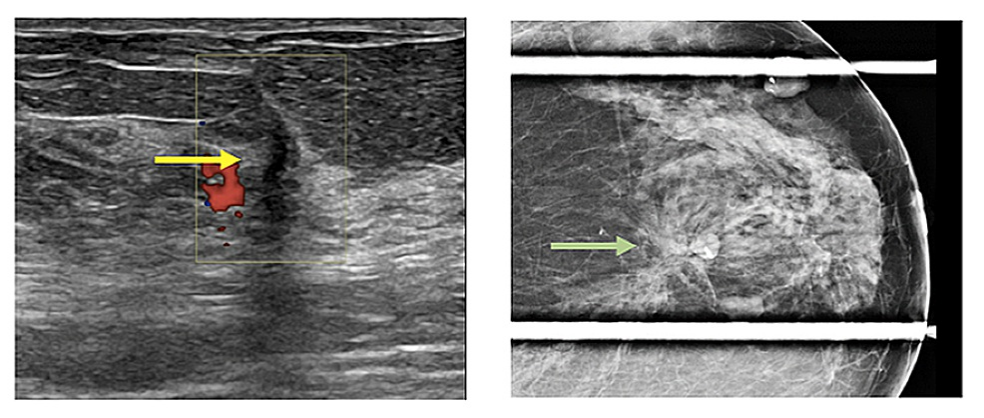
Pathology-proven papilloma with architectural distortion in mammogram (green arrow) and architectural distortion in ultrasound (yellow arrow).
